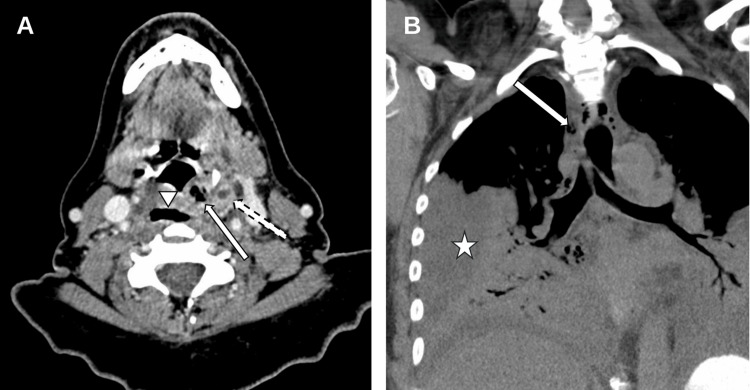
Initial CT imaging demonstrating descending necrotizing mediastinitis. (A) Contrast-enhanced axial CT neck shows extraluminal gas adjacent to the left hypopharynx/piriform sinus region (arrow) with retropharyngeal/prevertebral gas (triangle and dashed arrows). (B) Coronal CT chest shows pneumomediastinum (arrow) and pleural effusion (star).
